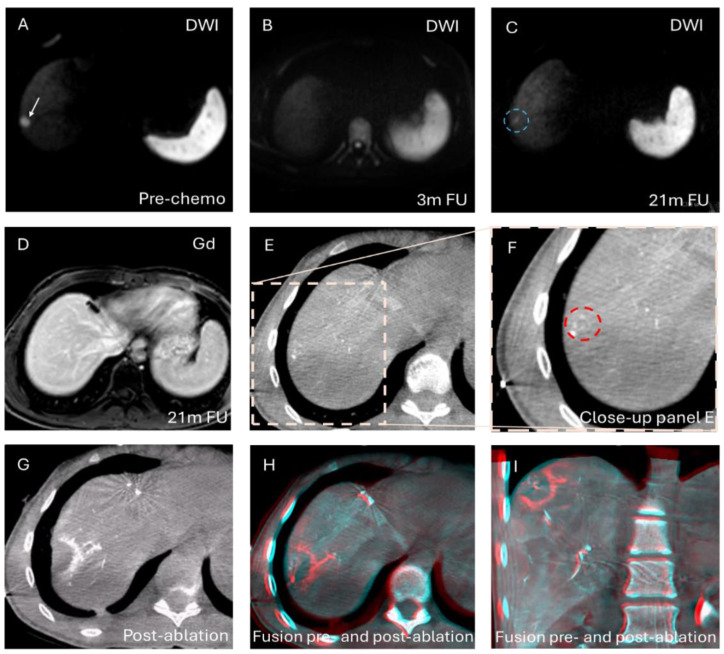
An example of an ablation of a reappearing CRLM that initially disappeared after systemic therapy induction (3 min at 75W). (A). The lesion is visible on DWI (white arrow) prior to chemotherapy (FOLFOXIRI-B) induction; (B). The lesion disappeared at 3 months follow-up (FU); (C). The lesion reappeared as a small diffusion-restricted focus (blue circle) at 21 months follow-up (size: 4 mm); (D). The same lesion is barely visible on gadolinium-enhanced MRI (Gd); (E,F). The lesion is identified on intraprocedural C-arm-CTHA (red circle); (G). The ablation zone depicted with C-arm-CTHA immediately after ablation; (H,I). Pre- and post-ablation C-arm-CTHAs are fused using the XperGuide software in the axial and coronal planes, showing adequate ablation margins.
